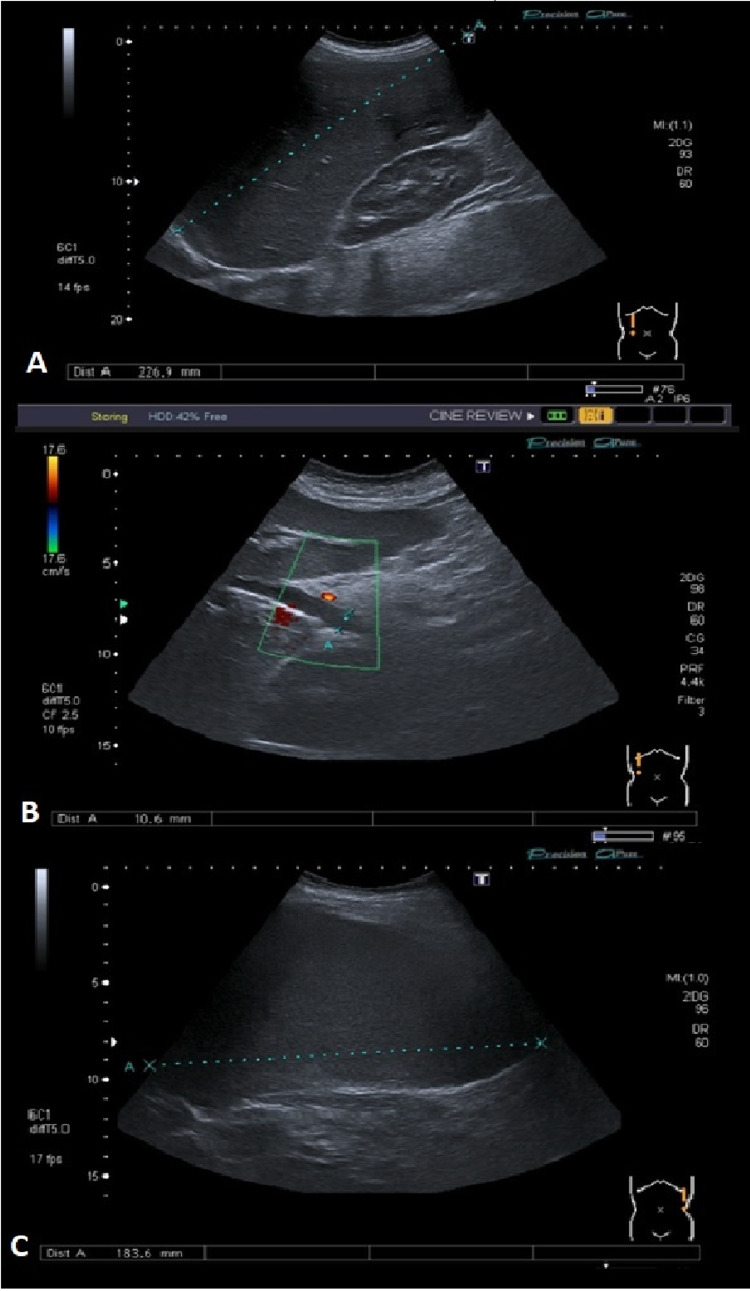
Abdominal ultrasound at ER admission A. Hepatomegaly (22.7 cm). B. Slight prominence of the intrahepatic biliary tract and main biliary ectasia (10 mm) without obstructive cause. C. Globular splenomegaly (18 cm) ER: emergency room
